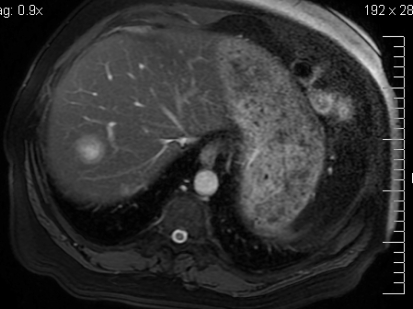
Magnetic resonance imaging of the abdomen, showing a mass in the right lobe of the liver. No lymphadenopathy or splenomegaly is seen.
